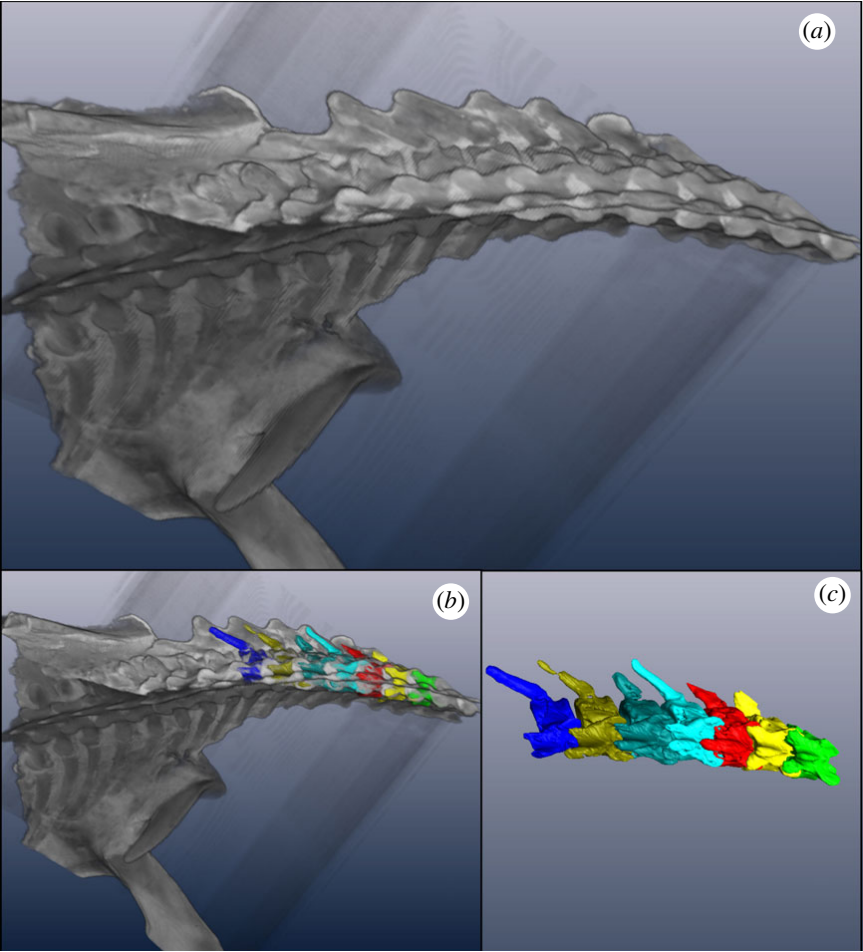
(*a*) Isosurface of *Nichollssaura borealis* produced in Amira 5.4 from CT scans, in dorsal view (dv). (*b*) Volumized cervical vertebrae (dv). (*c*) Isolated cervical vertebrae (dv), with isosurface removed. This process continues until all the cervical vertebrae are isolated out by volumization in Amira 5.4.
